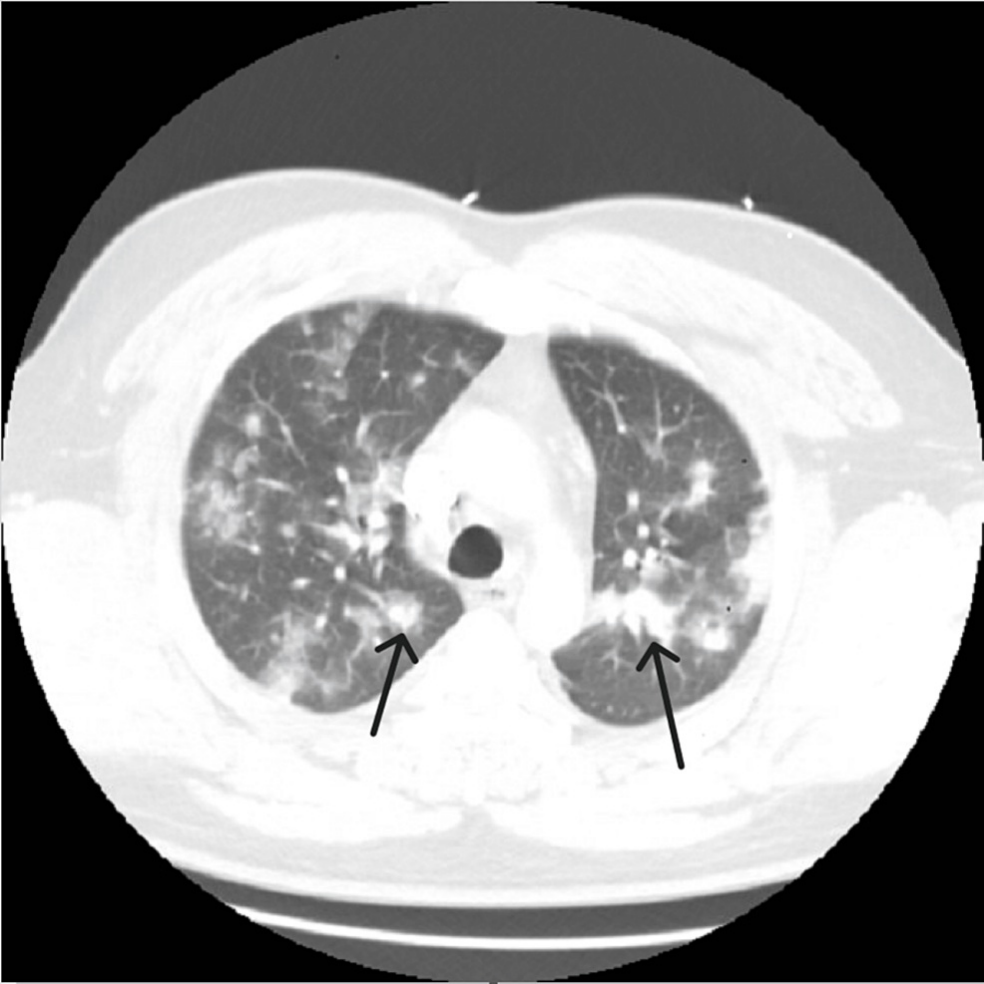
Chest computed tomography (CT) with ground-glass opacity, almost cannonball appearing (black arrows) in a case of acute eosinophilic pneumonia (AEP)
